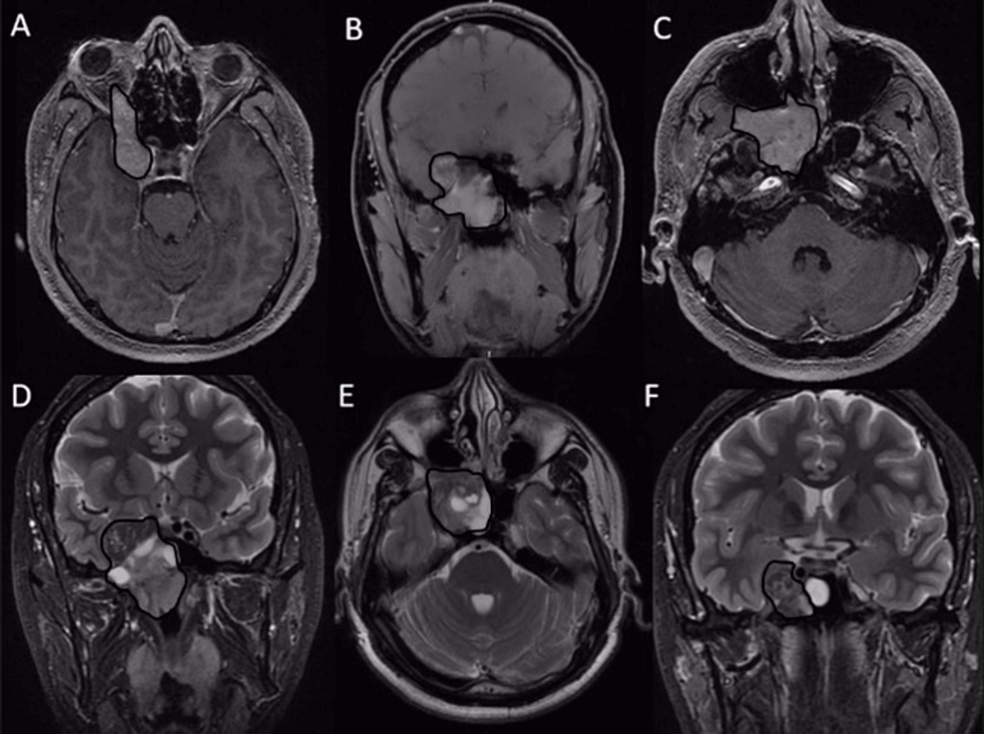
Preoperative brain MRI The nonencapsulated lobulated hypervascular lesion depicted in images 2A, 2B, and 2C can be observed extending from the right orbital fossa anteriorly to the right temporal fossa posteriorly and occupying the right cavernous sinus. In images 2D, 2E, and 2F, the characteristic MRI appearance of this entity is evident, with areas exhibiting "flow voids" (commonly referred to as "salt and pepper") [12] and some cystic foci visible in T2-weighted hyperintense images. The epicenter of this tumor is located in the right cavernous sinus, and it extends medially to the right sphenoid sinus and laterally to the middle cerebral fossa. MRI: magnetic resonance imaging
